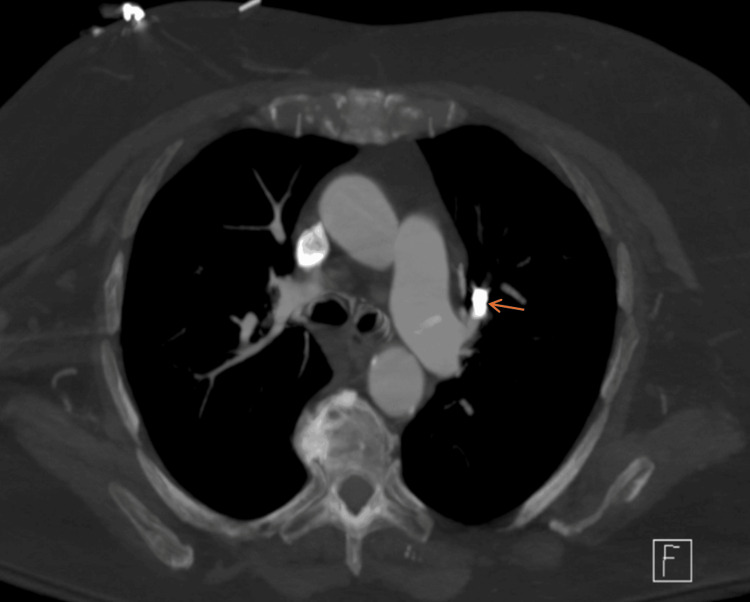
CT scan on the day of presentation showing a metallic foreign body within the left pulmonary artery (orange arrow).
